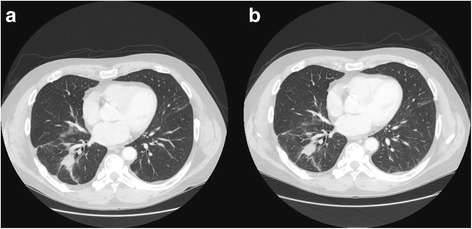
Chest CT Scans of Patient on Osimertinib Treatment. a Chest CT Scan Prior to Osimertinib Treatment. b CT Chest w/Abdomen wwo/Pelvis w Contrast Scan Post-Osimertinib Treatment
